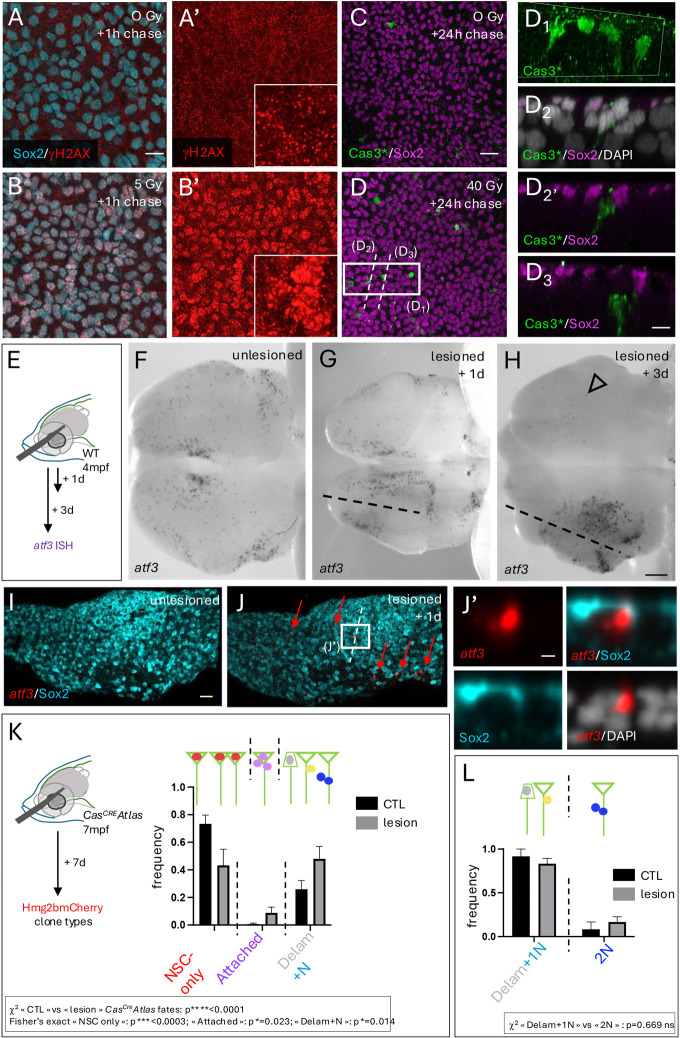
Differential recruitment of *atf3*^pos^ and Cas3* events under irradiation versus mechanical lesion. (A-D_3_) Effect of X-rays on NSCs *in vivo*. (A-B′) γH2AX DNA repair foci (red) in NSCs/NPs (Sox2^pos^, cyan) revealed by whole-mount IHC on adult pallia under control (A,A′) or irradiation conditions (B,B′) (5 Gy+1 h chase). (C-D_3_) Casp3* induction (green) in NSCs/NPs (Sox2^pos^, magenta) revealed by whole-mount IHC on adult pallia under control (C) or irradiation conditions (D) (40 Gy+24 h chase). D_1_ shows high magnification 3D rendering (Imaris) of the boxed area in D; D_2_, D_2_′ and D_3_ show sections across Cas3* cells as indicated in D. (E-L) Effect of mechanical lesion. (E-J′) *atf3* expression detected by chromogenic ISH (F-H: black; I-J′: red) on whole-mount pallia 1 day (G,I,J) or 3 days (H) following stab-wound injury (E) versus uninjured brains (F,I). Dorsal views, anterior left; one hemisphere is shown in I,J. Dashed lines indicate lesion trajectories; open arrowhead indicates downregulated *atf3* expression in the contralateral hemisphere at 3 dpl. (J′) High magnification section across an *atf3*^pos^ cell along the dashed line in J. (K) Proportion of *Cas^CRE^Atlas* clone types in control versus lesioned hemispheres at 7 dpl. Graph displays mean±s.e.m. Statistical analysis for global differences between *Cas^CRE^Atlas* clone types between lesioned and unlesioned hemispheres: contingency χ^2^ test: *P*<0.0001; statistical analyses for individual fates among three possible fates [NSCs (NSC singlets and doublets), attached clones, and neurogenic clones (delaminating cells, 1N or 2N; this category contains >70% 1N clones in both cases)]: Fisher's exact test with Bonferroni correction: NSC P*<0.0003; attached: P*=0.023; Delam+N: P*=0.014. Control: 8 hemipallia, *n*=84 clones; Lesion: 7 hemipallia, 79 clones. (L) Proportion of neurogenic *Cas^CRE^Atlas* clone types in control versus lesioned hemispheres at 7 dpl, distinguishing single versus double neurons. Graph displays mean±s.e.m. Statistical analysis for global differences between single versus double neurons: contingency χ^2^ test: *P*=0.669. CTL, control; ns, not significant. Scale bars: 10 µm (A,A′,B,B′); 20 µm (C-C′); 10 µm (D1-D3); 100 µm (F-H); 30 µm (I,J); 10 µm (J′).
